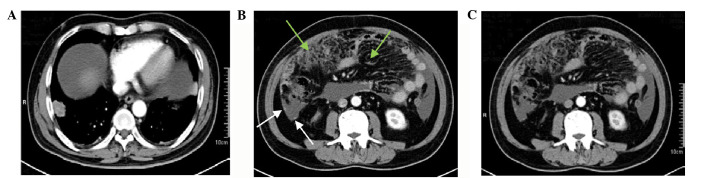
Computed tomography (CT) of the lungs and abdomen. (A) Primary lesion in the lower lobe of the right lung. (B and C) Caked colic omentum thickening (green arrow) and nodule-like thickening (white arrow) was more evident in the vein phase (B) than in the artery phase (C), as observed by CT with contrast enhancement.
